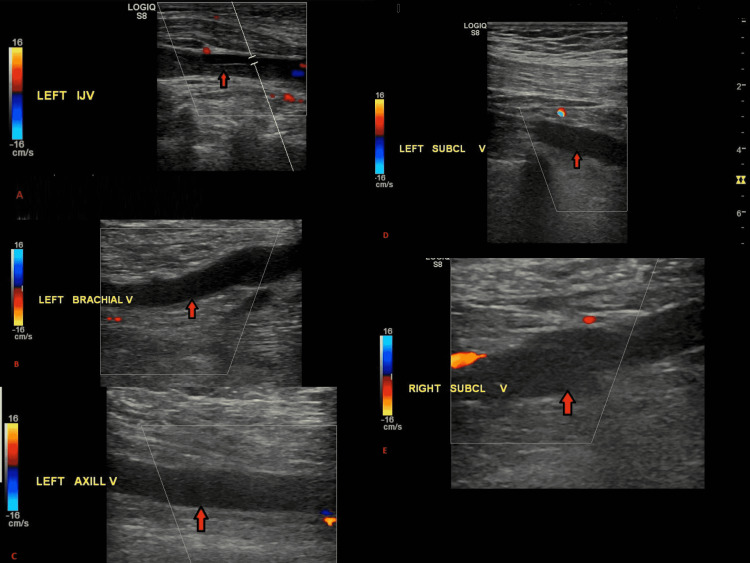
Venous duplex ultrasound showing clots in the left internal jugular (A), left subclavian (B), left axillary (C), and (D) left brachial veins. A clot can also be seen in the right subclavian vein (E).
